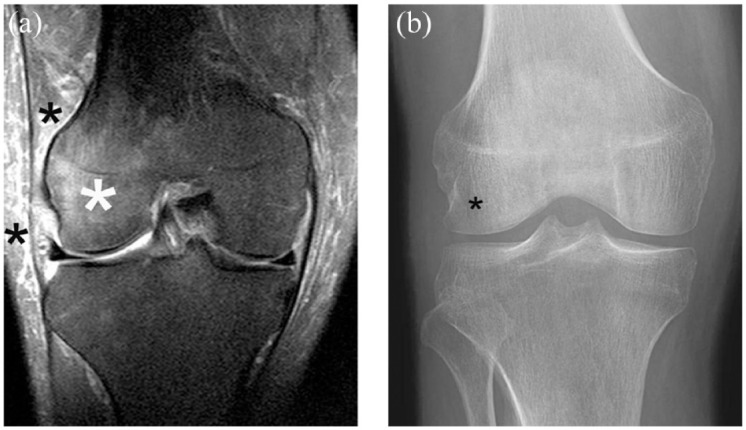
Discordant pain to X-ray. Severe pain in a radiographically normal joint at screening should alert the investigator to further work. Also a sudden increase in pain during the course of the study should result in further exploration regarding the cause of symptom worsening. In this case, primarily so-called for-cause radiographs would be obtained but these may be unremarkable. Additional for-cause MRI is the next step in order to visualize potential x-ray occult structural findings. (a) The image example (coronal fat-suppressed proton density-weighted MRI) show an extensive bone marrow edema alteration in the lateral femoral condyle (large white asterisk). Finding is consistent with idiopathic bone marrow edema syndrome, a disease entity that is strongly associated with severe pain, may be self-limited and heal without sequelae or progress to SIF and structural deterioration. Note additional soft tissue changes between the iliotibial band and in the lateral subcutaneous soft tissues (small black asterisks). These soft tissue alterations are a common accompanying finding. (b) The corresponding radiograph was considered normal at the time of centralized reading. Retrospectively, a subtle diffuse osteopenia in the lateral condyle compared to the medial side may be noted (asterisk). Prior the era of MRI the disease entity was labeled as transient osteoporosis for this reason.
